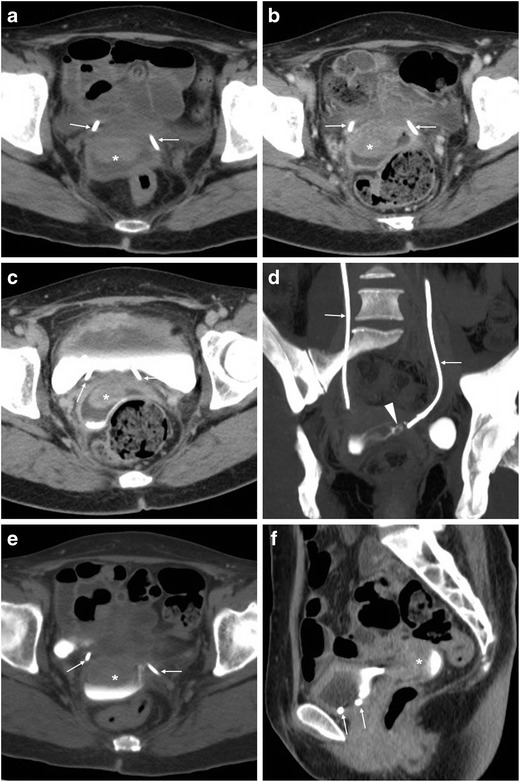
Another case of ureteral injury shortly after LH. Despite preoperative bilateral ureteral stenting (thin arrows), unenhanced CT images (A) showed a mixed-fluid and hyperattenuating pelvic collection (*), with corresponding peripheral enhancement in the venous phase (B) and filling by opacified urine in the excretory phase (C). On additional delayed acquisition, leakage from the most distal tract of the left ureter (arrowheads in D, E) and progressive opacification of the urinoma (*) were seen. Endourological treatment was performed (Partially reproduced from open access ref. no [58])
